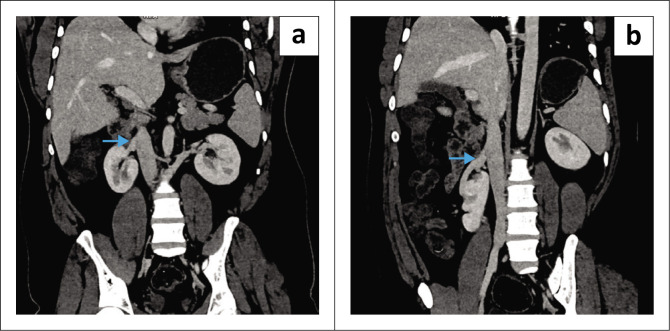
(a) Venous phase contrast-enhanced CT coronal reconstruction revealing the renal vein (blue arrow) draining the cranially placed right kidney joining the inferior vena cava. (b) Coronal reconstruction showing a second renal vein (blue arrow) draining the caudally placed right kidney to the inferior vena cava.
